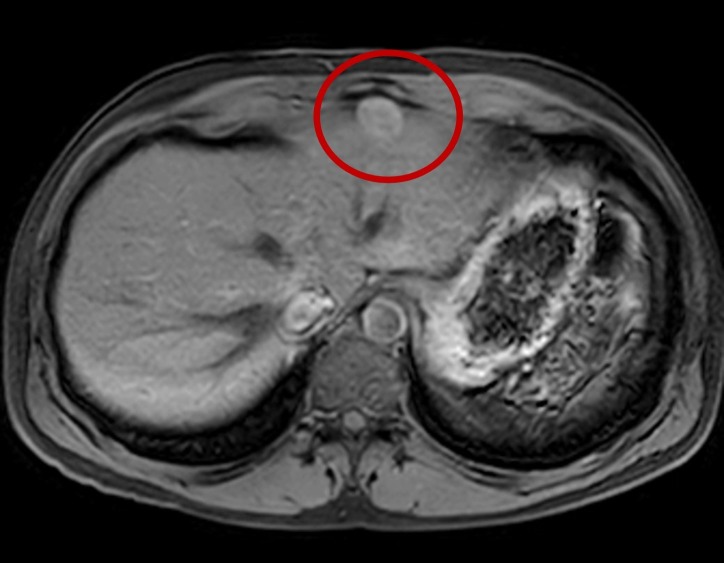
Magnetic resonance imaging (MRI) abdomen showing a mass (red circle) in segment 2 of the liver measuring 2.4 x 2.0 cm arising exophytically and anteriorly, similar to that seen in the previous computed tomography (CT) abdomen.
